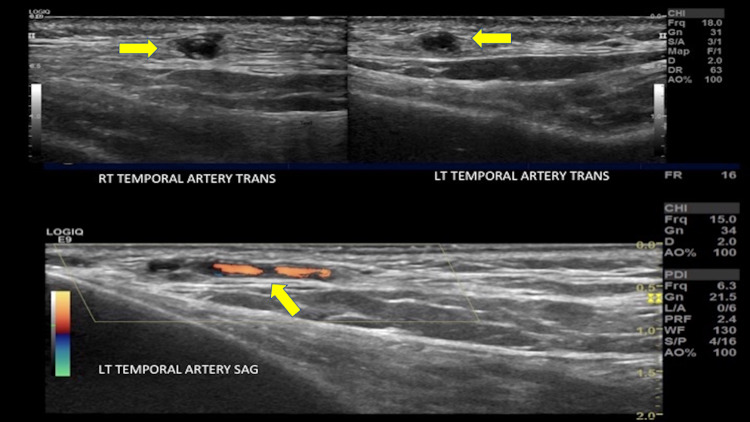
Ultrasound Doppler of Temporal Arteries demonstrating bilateral hypo-echoic thickening known as "Halo sign" (yellow arrowheads) suggestive of Temporal Arteritis.
